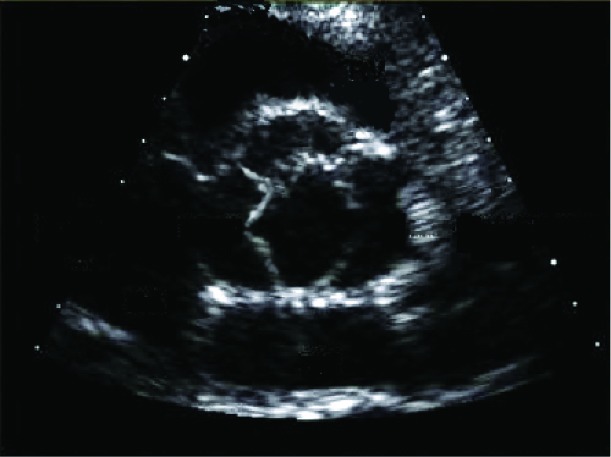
Variables for analysis included age, gender, echocardiographic measurements of both atria and ventricles, grades of left ventricular diastolic function, aortic valve phenotype, pattern and severity of valvular dysfunction and associated aortopathy. Phenotypes of BAV and aortopathy classifications used in this study are given in Table 1.^11^
